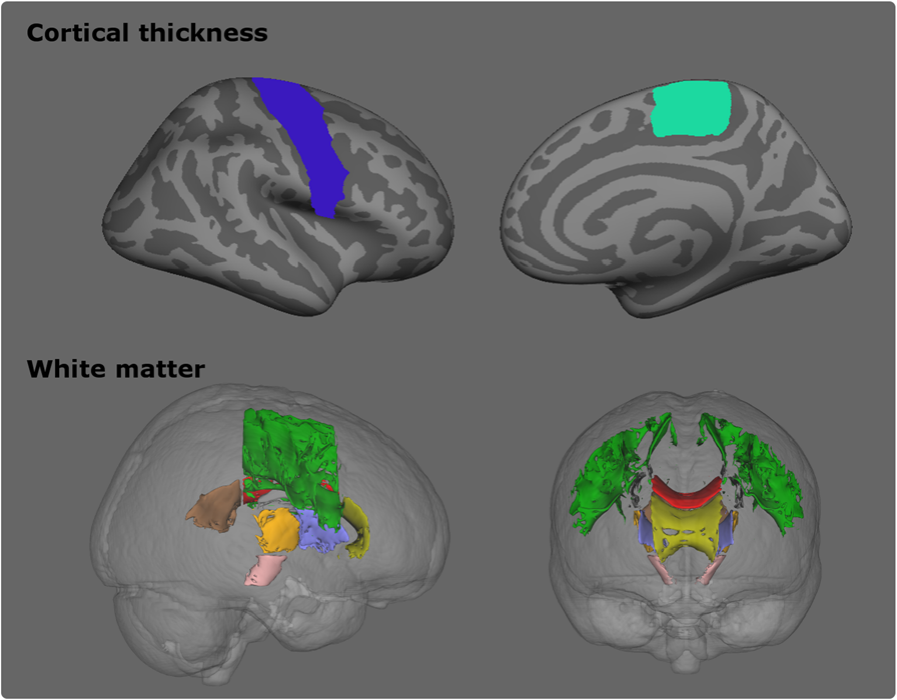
MRI features included in the regression analyses. *Top*: Cortical thickness. *Blue*: precentral gyrus, *Light green*: paracentral gyrus. Only the *right* hemisphere is displayed. *Bottom*: *White* matter. *Green*: superior corona radiata, *grey*: corona radiata, *orange*: posterior limb of the internal capsule, *lilac*: anterior limb of the internal capsule, *rose*: cerebral peduncle, *yellow*: genu of corpus callosum, *red*: body of the corpus callosum, *brown*: splenium of the corpus callosum
